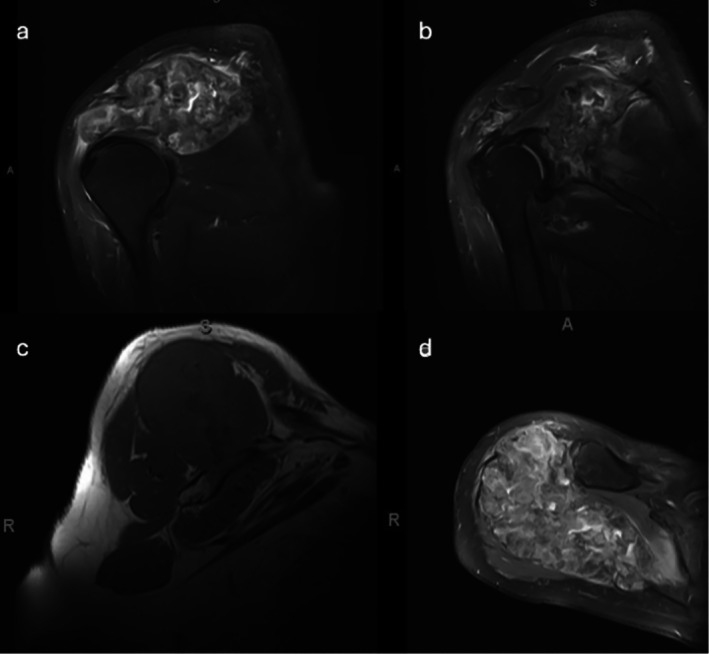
(a) Coronal T2 weighted MRI, (b) coronal T2 weighted MRI, (c) sagittal T1 MRI, and (d) axial T2 MRI of the right scapula all depicting a large heterogenous scapular mass with extensive soft tissue component and bony destruction. It also demonstrates lesion progression near the glenoid neck and in close proximity to the articular surface of the glenohumeral joint. T2 images also depict extensive inflammatory changes due to the reaction of the soft tissue as well as the resultant mass effect from the tumor.
